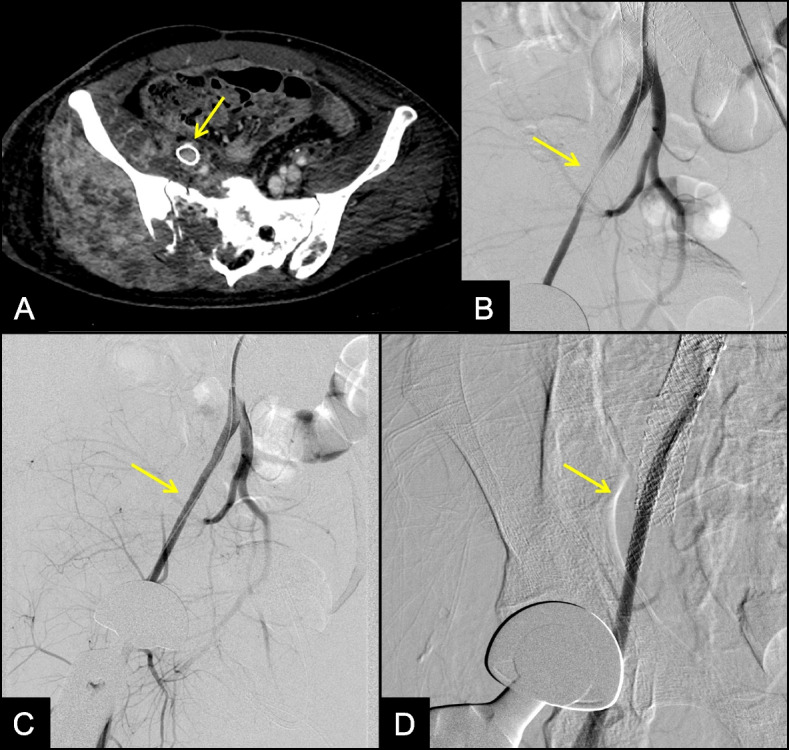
A Axial CT angiogram demonstrates high gradestenosis of the external iliac artery adjacent to the newly placed external iliac vein stent (arrow). B Angiogram through a 7 French sheath within the right common iliac artery demonstrates high grade narrowing of the external iliac artery secondary to compression from the adjacent venous stent (arrow). C and D Angiogram after placement of two overlapping 5 mm Viabahn VBX stents demonstrates resolution of the stenosis with brisk flow (arrows)
